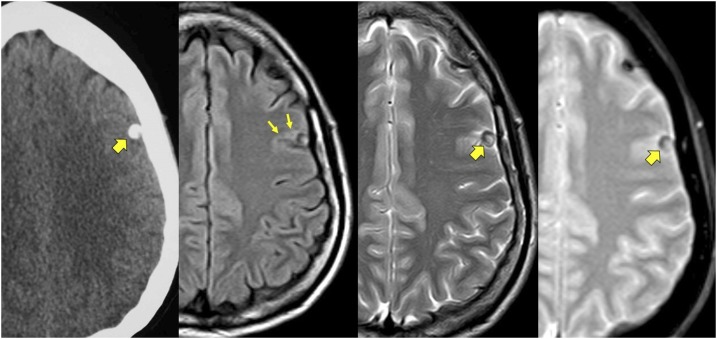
Left panel: Computed tomography showing a superficial single calcified cysticercus in the lower left frontal lobe (thick arrow). Left-central panel: fluid attentuated inversion recovery magnetic resonance imaging sequence showed the calcification surrounded by mild perilesional edema (small arrows). Right-central panel: T2-weighted sequence showing the calcification to be located in a cortical sulcus; the interior of the calcification is heterogeneous because of the presence of scolex remnants (thick arrow). Right panel: Echo-gradient sequence confirming the presence of scolex remnants within the calcification (thick arrow) and showing another solid calcification in the upper ipsilateral frontal lobe. This figure appears in color at www.ajtmh.org.
